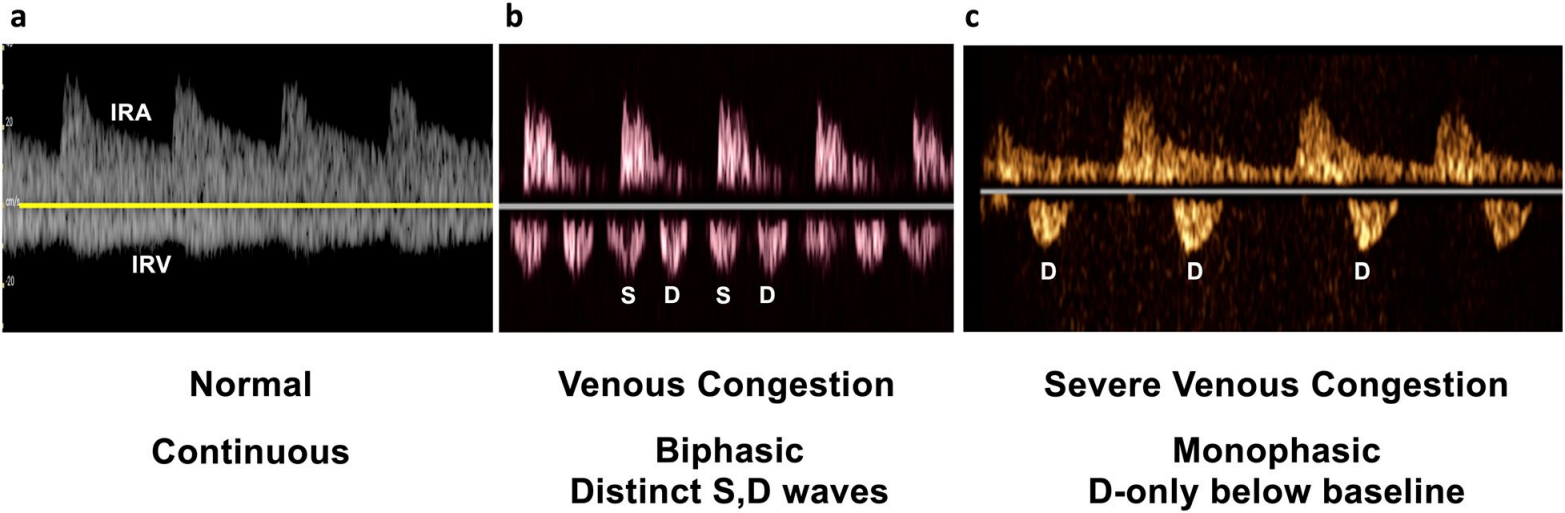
a Under normal conditions, the IRVD exhibits a continuous pattern with minimal pulsatility and no interruptions. b However, mild to moderate venous congestion results in heightened pulsatility and interruptions in the waveform, characterized by distinct systolic (S) and diastolic (D) waves. c In severe congestion, S-reversal occurs similarly to that of the HV, leaving only the D-wave below the baseline. IRVD, intrarenal vein Doppler; HV, hepatic vein; IRA, intrarenal artery; IRV, intrarenal vein
